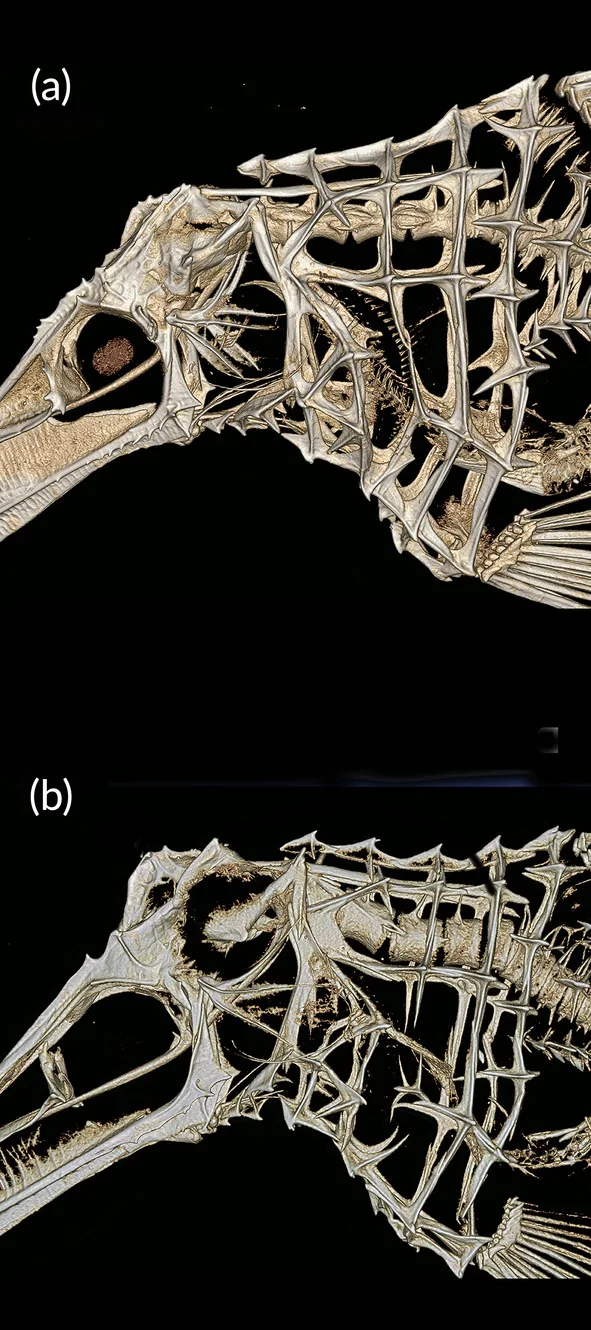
Micro‐CT reconstructions of the pretrunk and supraoccipital crest of *S. snuffleupagus*: (a) holotype, female, AMS I.33751‐047; (b) paratype, male, NTM S.13600‐047.
